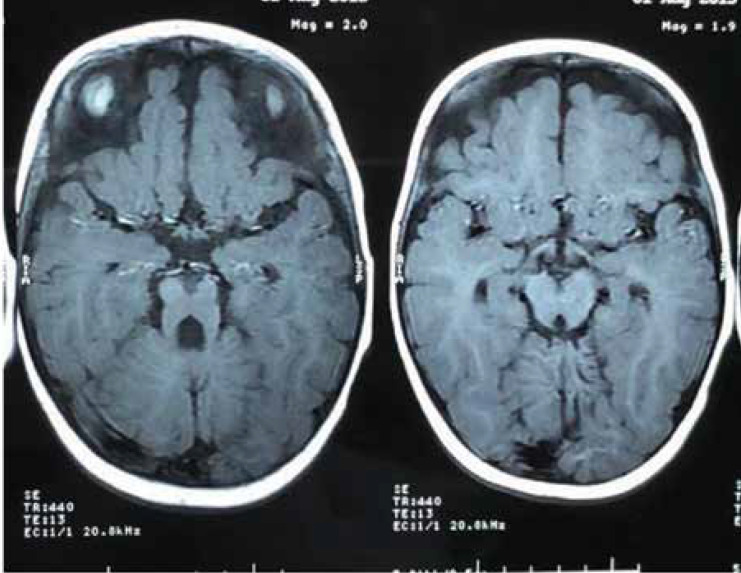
Brain magnetic resonance image (MRI) of the patient indicating the characteristic molar tooth sign
